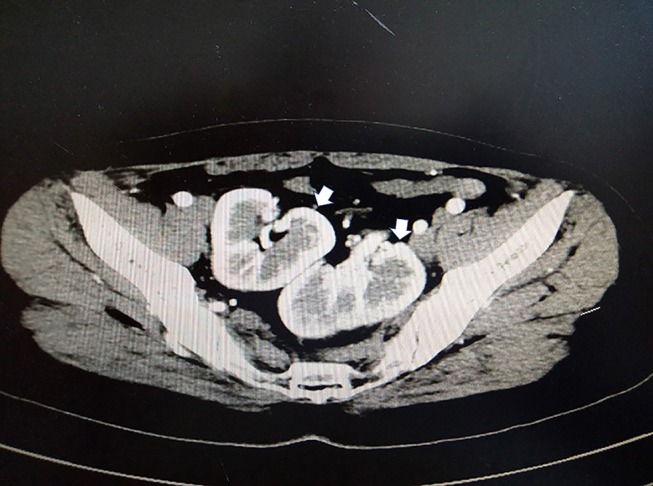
Contrast-enhanced CT presenting bilateral kidneys located in the pelvic cavity (white arrow).
